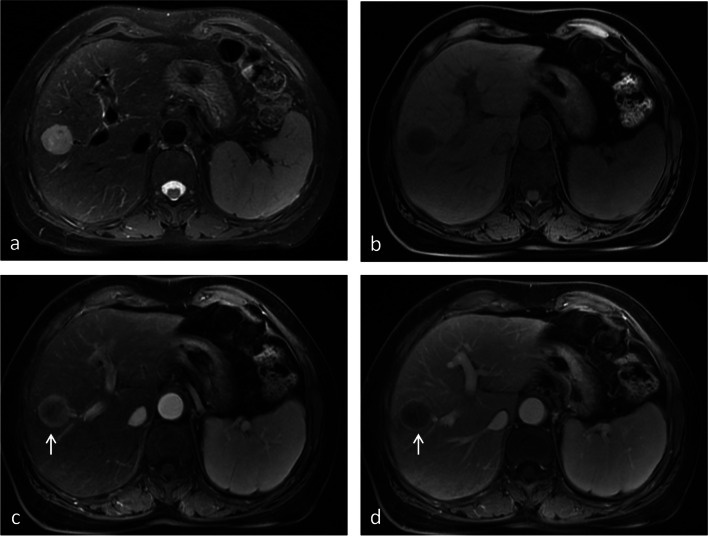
Images from a 66-year-old female with CRLM. a The T2-weighted image and (b) T1-weighted image show a 2.5-cm lesion in segment VIII of the liver. c The arterial phase image shows rim enhancement of the lesion (white arrow). d The portal venous phase image shows peripheral washout and continuous rim enhancement (white arrow). Hepatic capsular retraction, peripheral hepatic enhancement, vessel penetrating the tumor, and upper abdominal lymphadenopathy are not shown. Thus, a score of 6 was assigned for this patient
